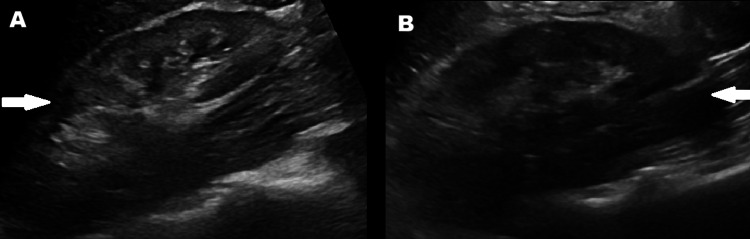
Ultrasound of the right kidney (A) and left kidney (B) showing normal ultrasound appearance (arrows).
